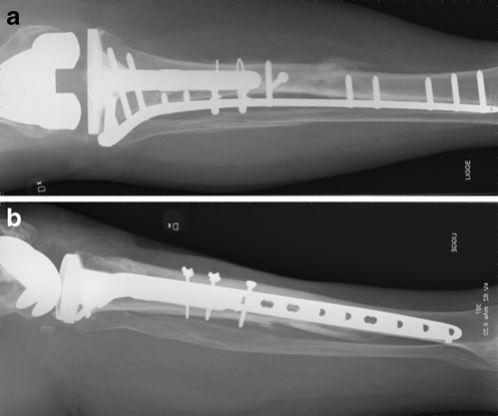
a, b Postoperative X-ray 6 months after stabilisation with a locking plate (LISS; Synthes®)
